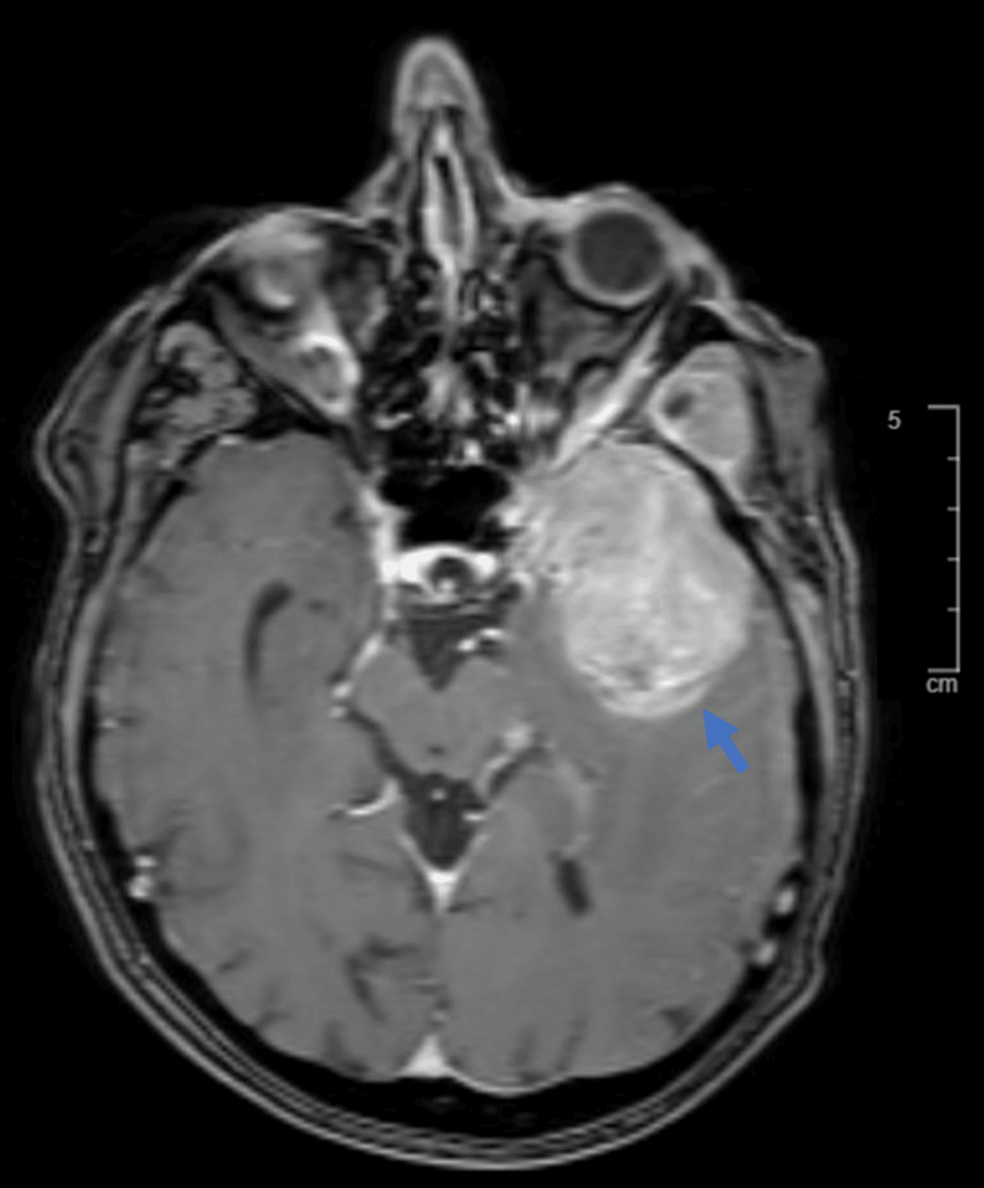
MRI scan of the brain metastasis. T1 MRI scan showed a left middle fossa brain mass (5.2 cm, blue arrow). MRI: magnetic resonance imaging.
